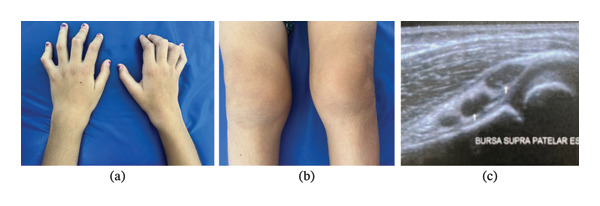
Patient 2: (a) camptodactyly with bilateral finger flexion and bilateral wrist swelling; (b) bilateral knee swelling; (c) knee ultrasound showing debris in the suprapatellar bursa.
